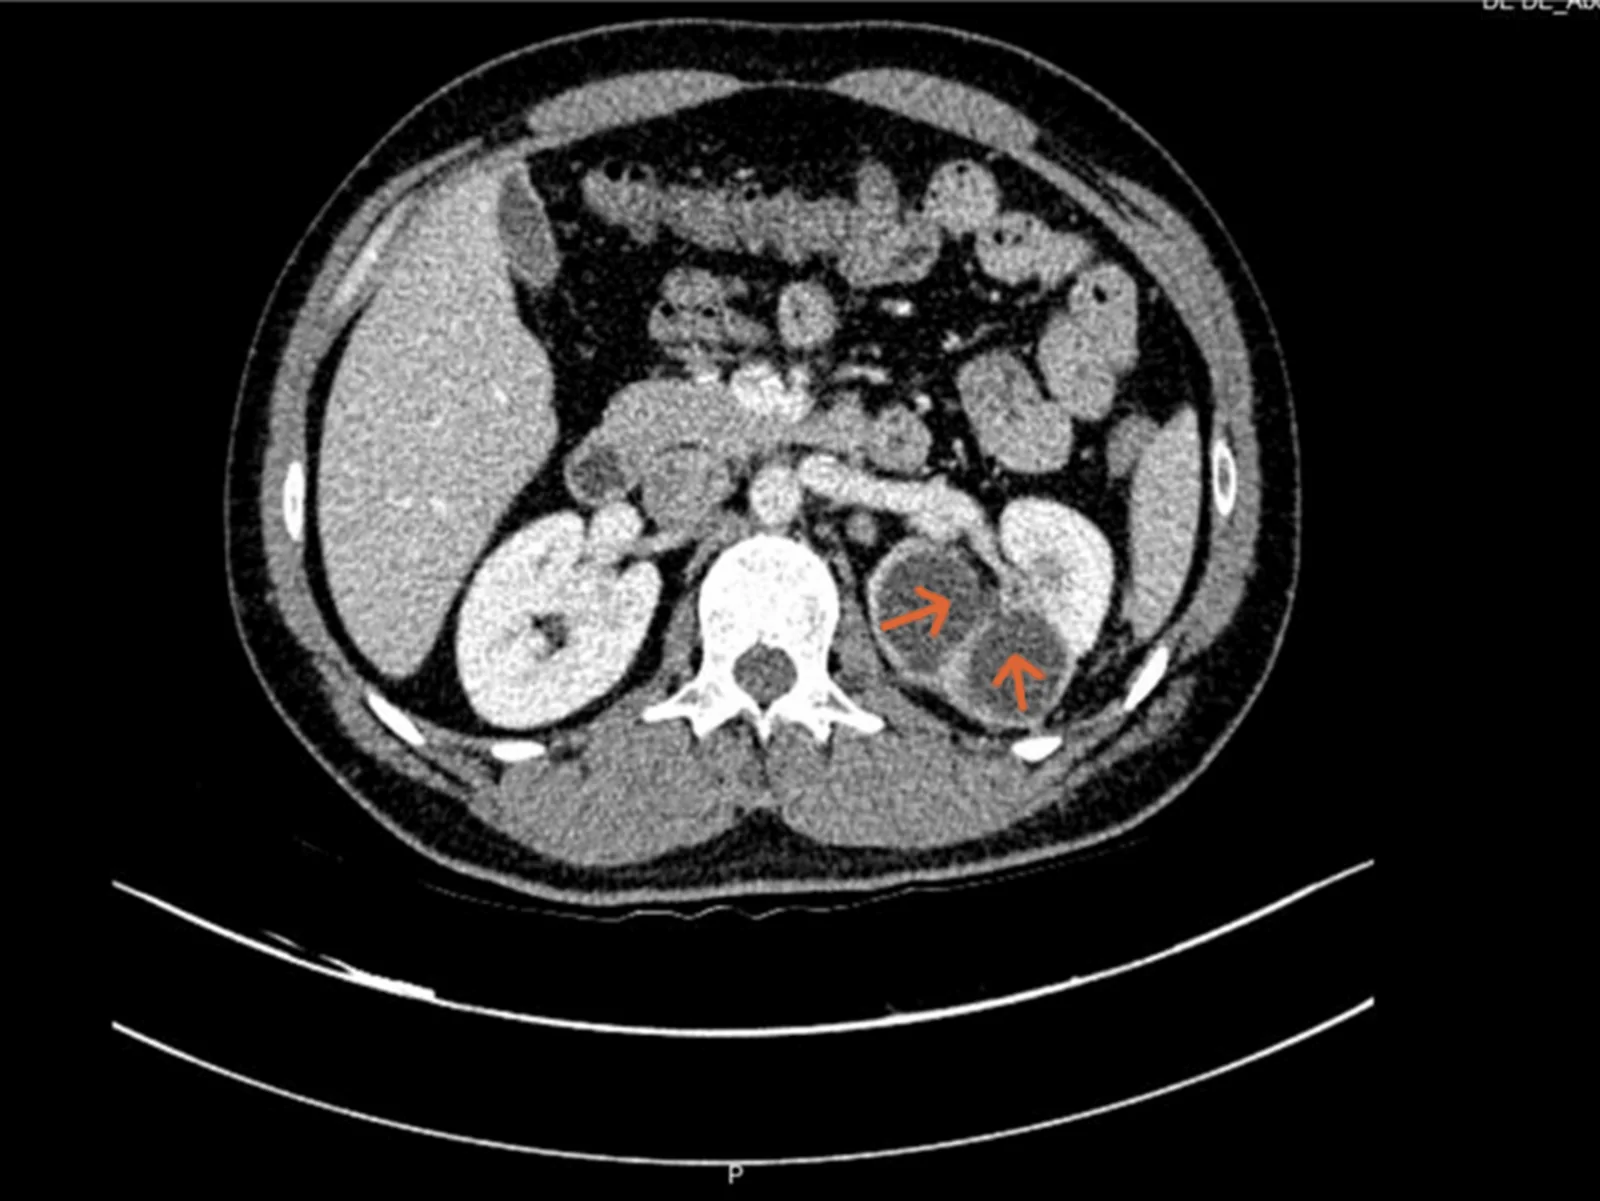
CT urogram showing calyceal neck stricture in left kidney (arrows) of case 2.
